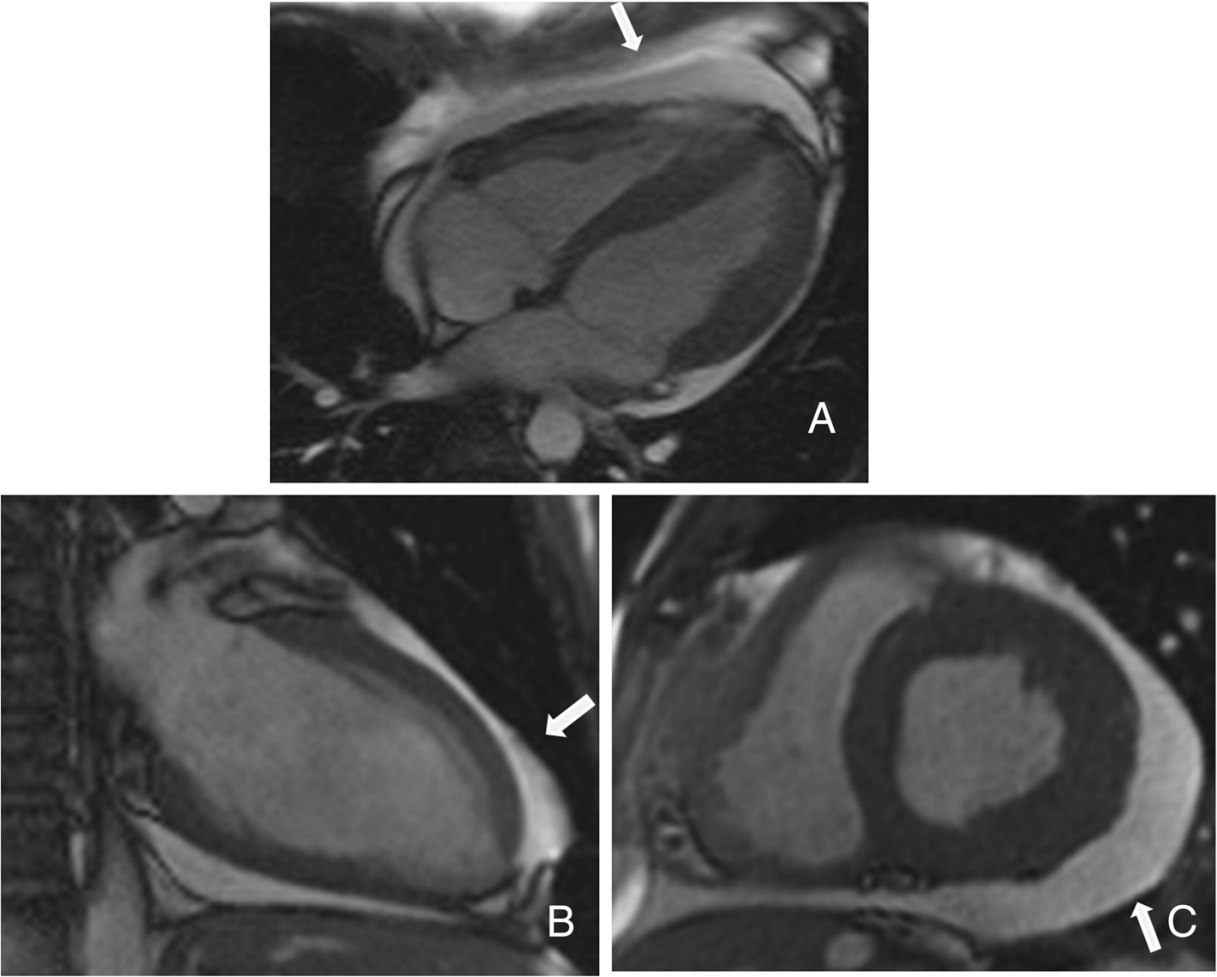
Cardiac magnetic resonance imaging (MRI), balanced steady-state free precesion (bSSFP) sequence. (A-C) These images show pericardial effusion of 1.2 cm.
